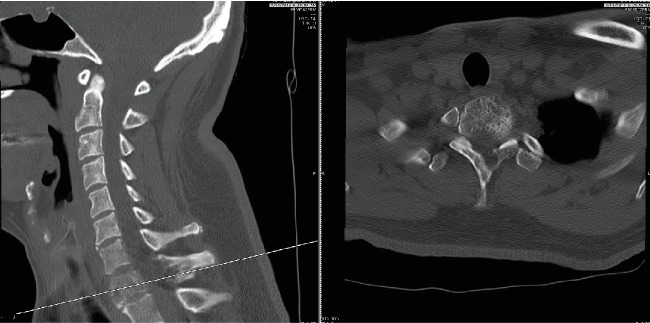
Preoperative computed tomography scan showing sagittal and axial views.
